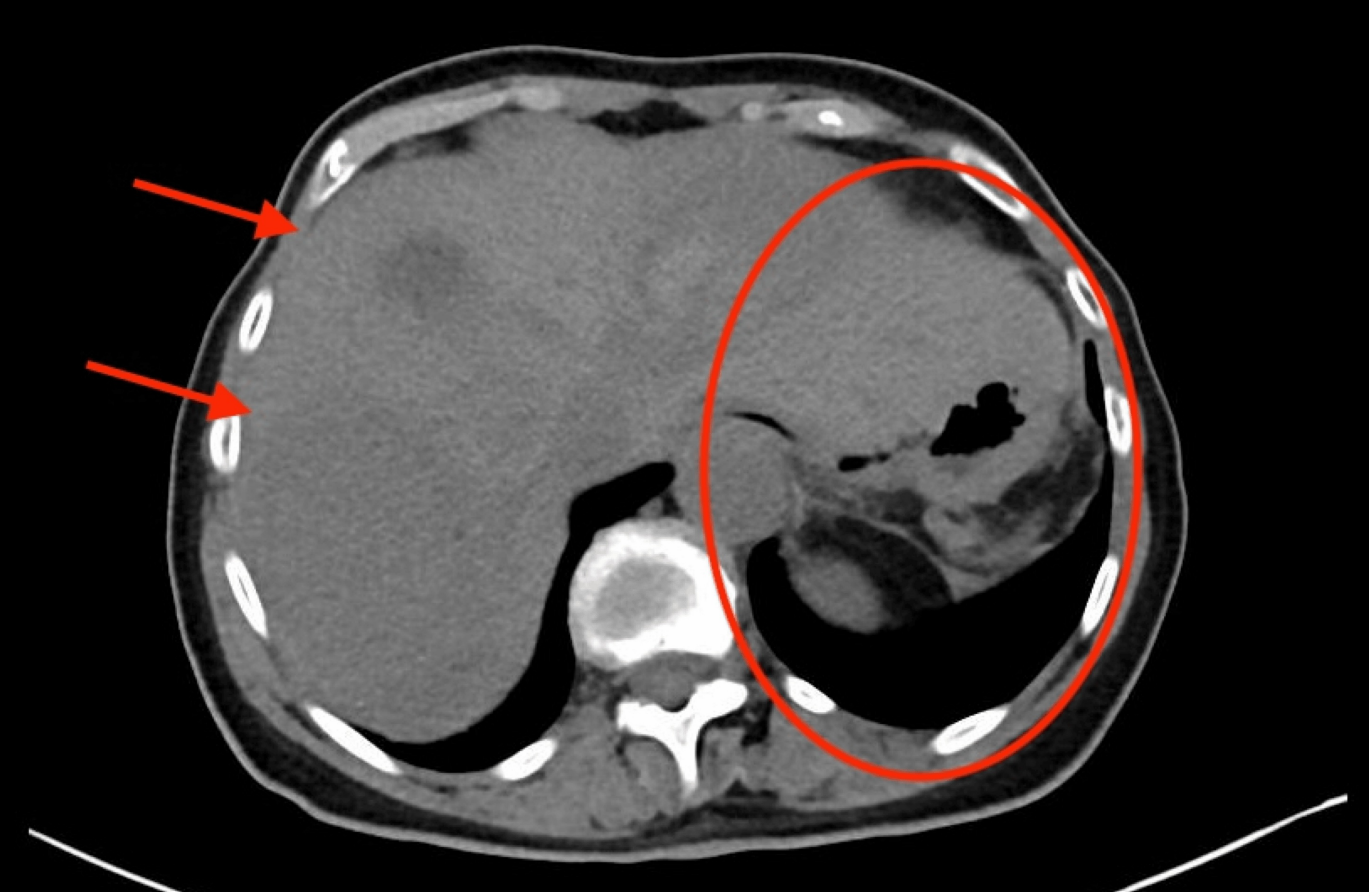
CT abdomen/pelvis post-splenectomy, showing evacuation of hemoperitoneum. Red arrows indicate post-operative changes. Red circle marks the splenectomy bed.
